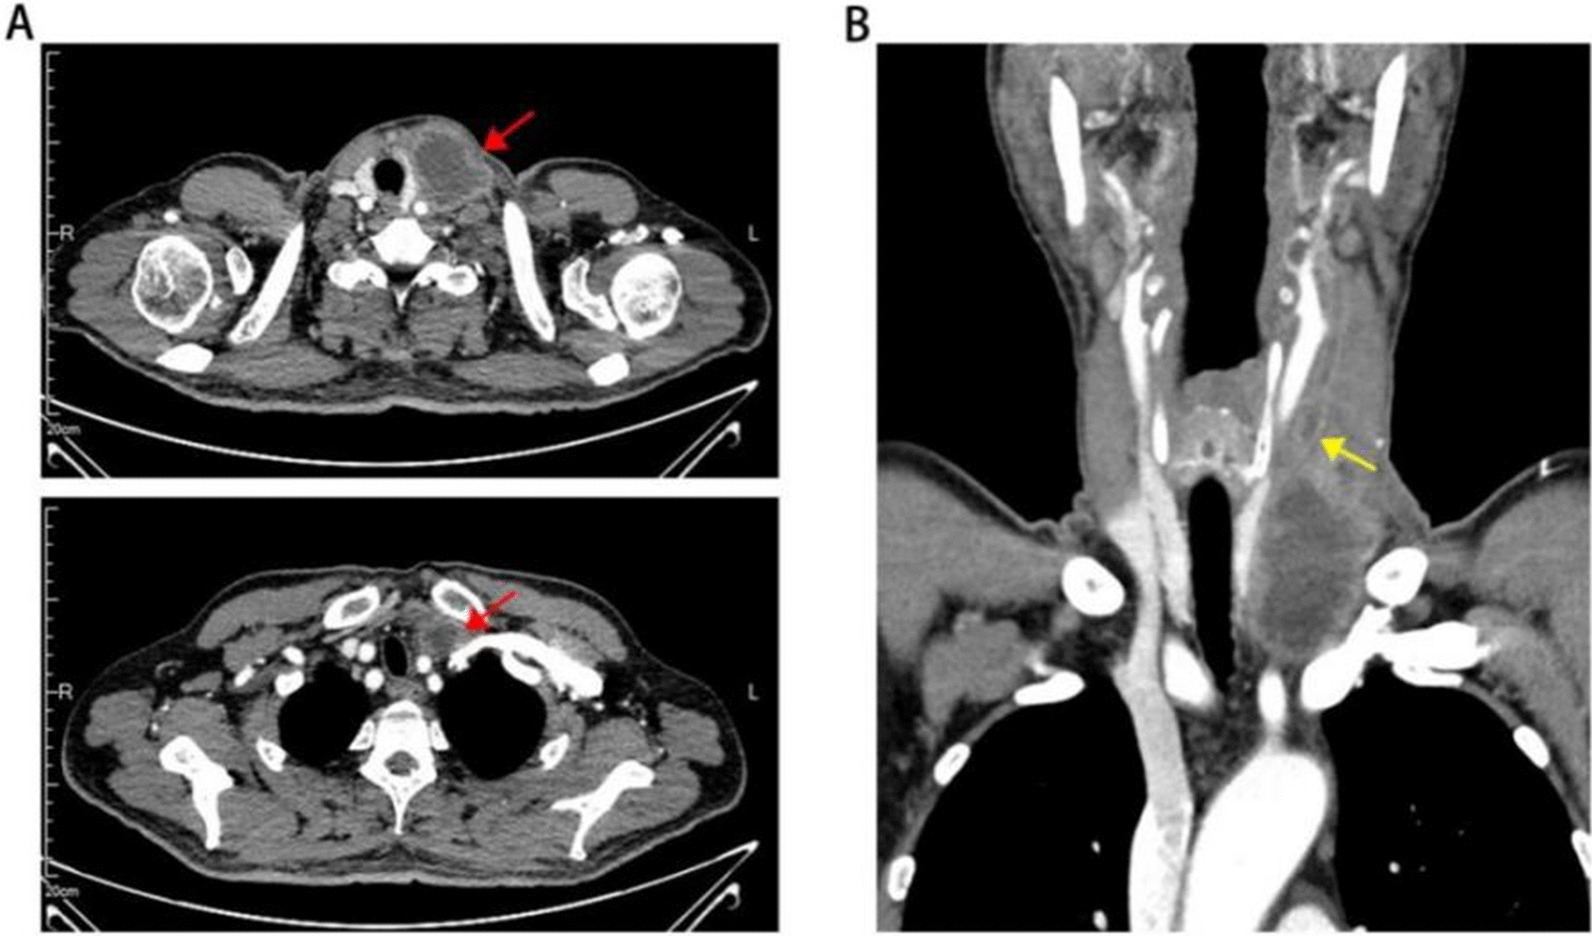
A Coronal plane of computed tomography showed neck mass spread down to superior mediastinum with iquefactive necrosis in the center (red arrows). Trachea was oppressed to the right side. B Significant intraluminal filling defect and thrombosis was found in internal jugular vein (yellow arrow)
